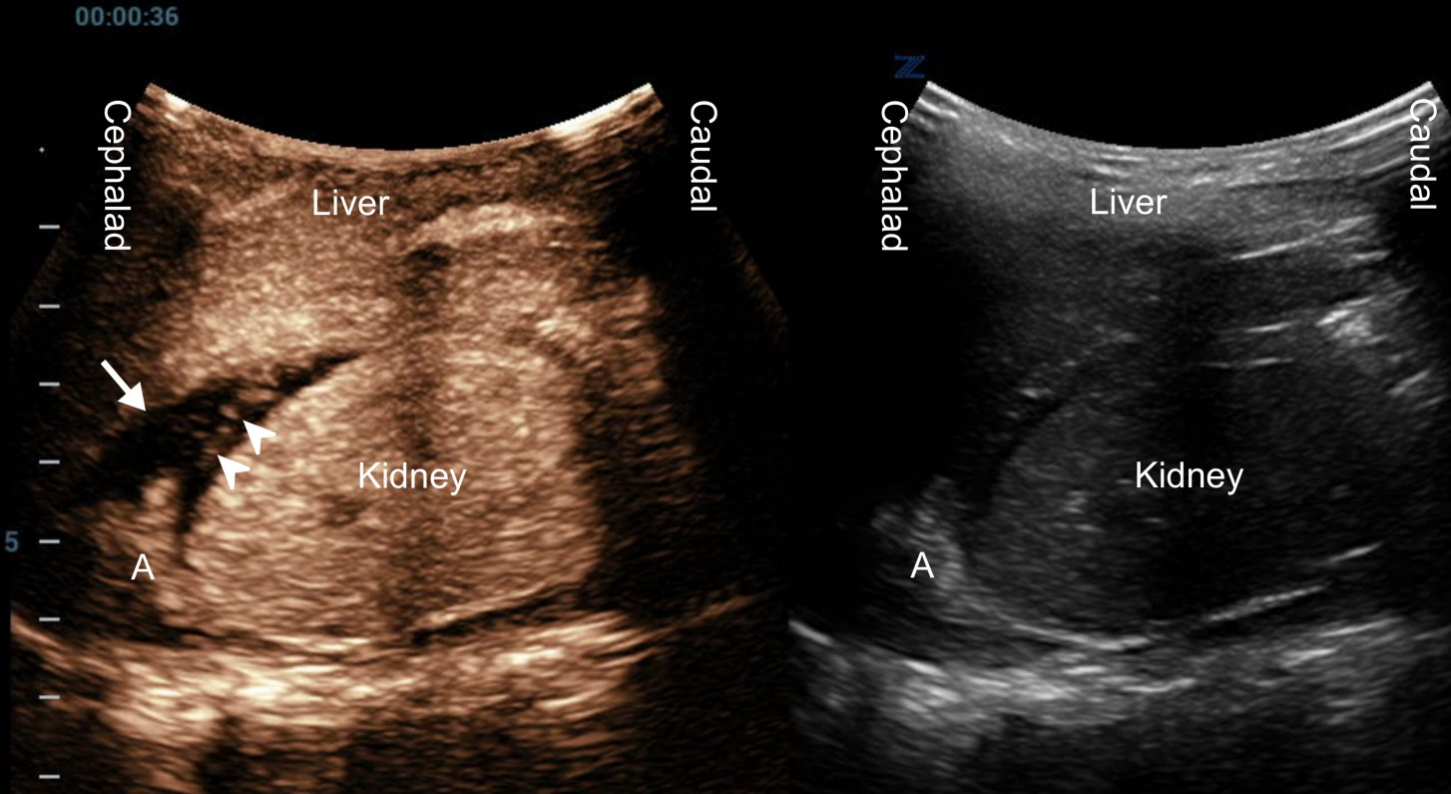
A dual-mode contrast-enhanced ultrasound (CEUS) image of the same (Figure 1) focused assessment with sonography in trauma (FAST) right upper quadrant (RUQ) view (left side - CEUS; right side - grayscale) demonstrating adrenal (A) bleeding. The hematoma appears non-enhancing hypoechoic (arrow), and the extravasation of bubbles (arrowheads) indicates active bleeding.
